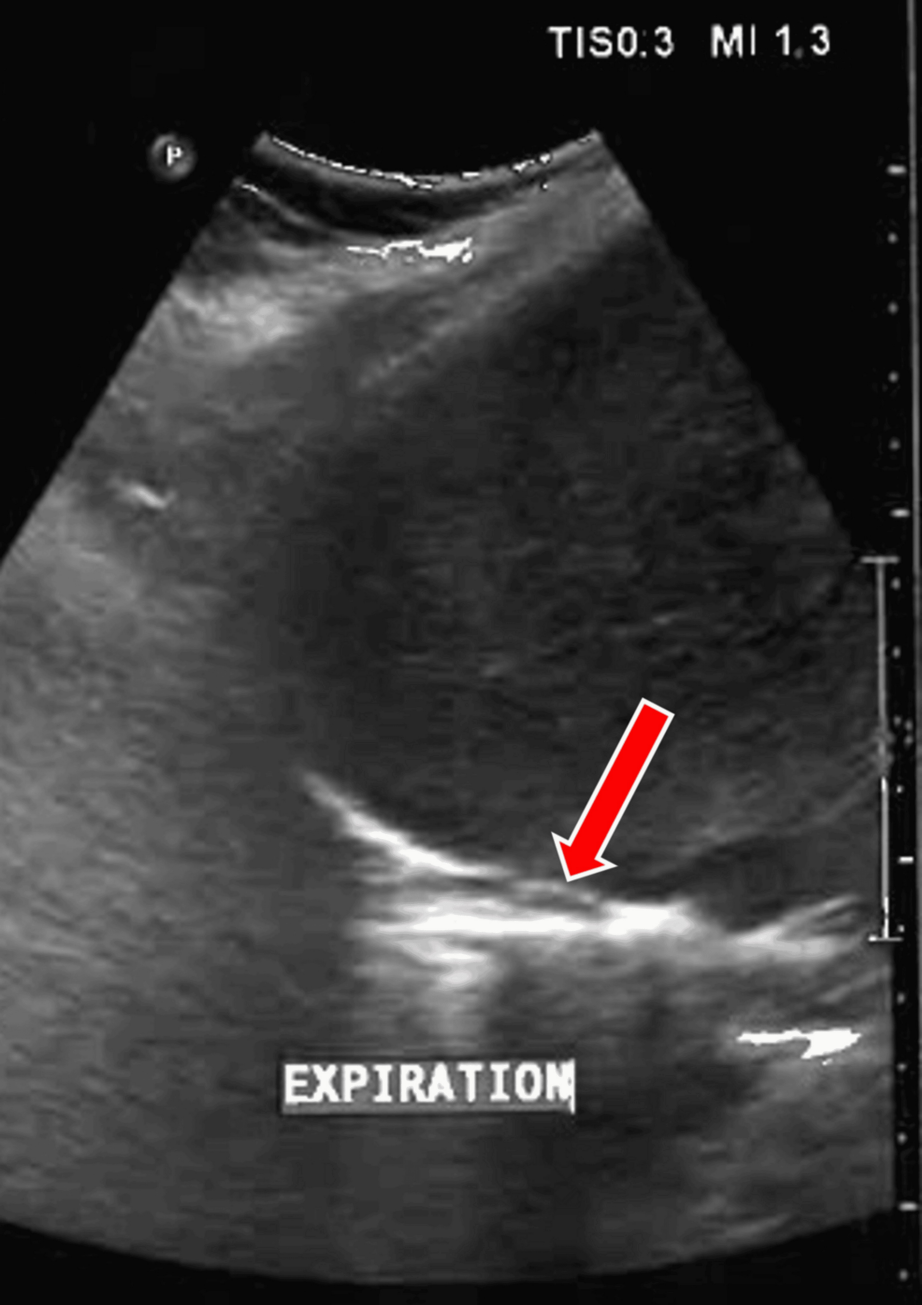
Ultrasonography of the abdomen and thorax portraying right diaphragmatic palsy. The red arrow denotes the diaphragm that shows no movement on expiration.
